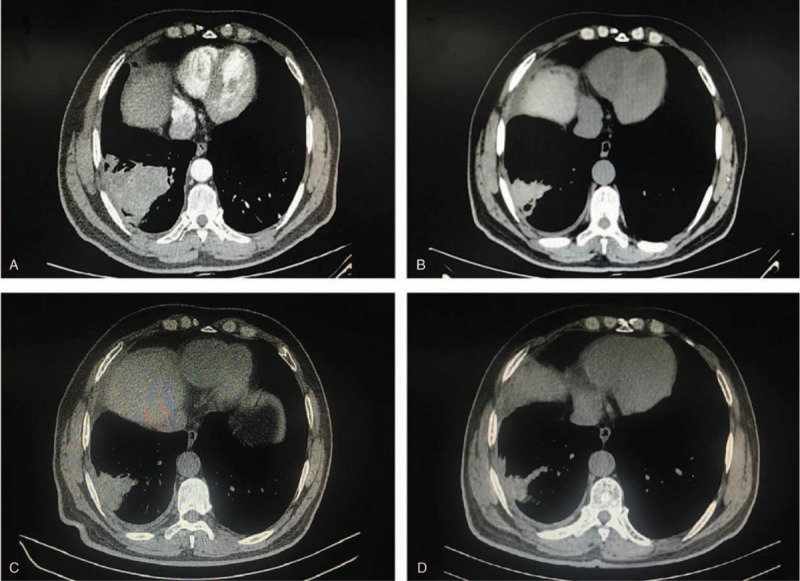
Radiographic findings obtained before and after sintilimab therapy. (A) Shows a 6.4×5.3 cm mass with heterogeneous density and irregular margins in the right lower lung on enhanced chest computed tomography before sintilimab therapy. (B) Shows markedly decreased size of right lower lung mass after 6 cycles of sintilimab on chest computed tomography. (C) Shows unchanged right lower lung mass size before durvalumab therapy. (D) Shows further regression of right lower lung mass size after 12 cycles of durvalumab on chest computed tomography.
